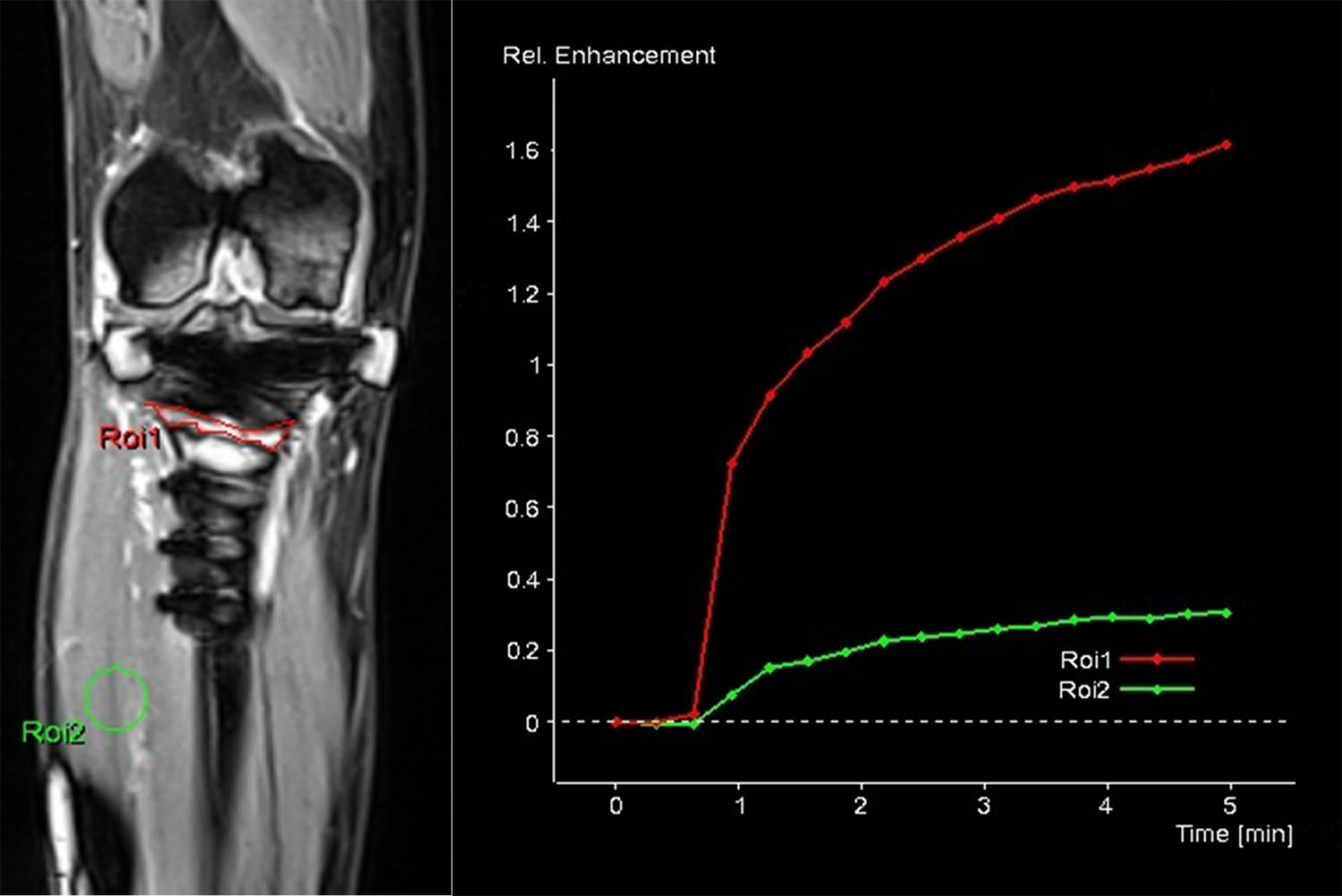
Dynamic magnetic resonance imaging (DCE-MRI) examination and evaluation of an osteotomy gap (patient without ABG). Left: DCE-MRI of the osteotomy gap. Right: evaluation over time of both regions of interests (ROI) with the dedicated quantification software Tissue4D (Siemens Healtcare). Red: ROI 1 in the osteotomy gap. Green: ROI 2 in adjacent muscle tissue (colour figure online)
